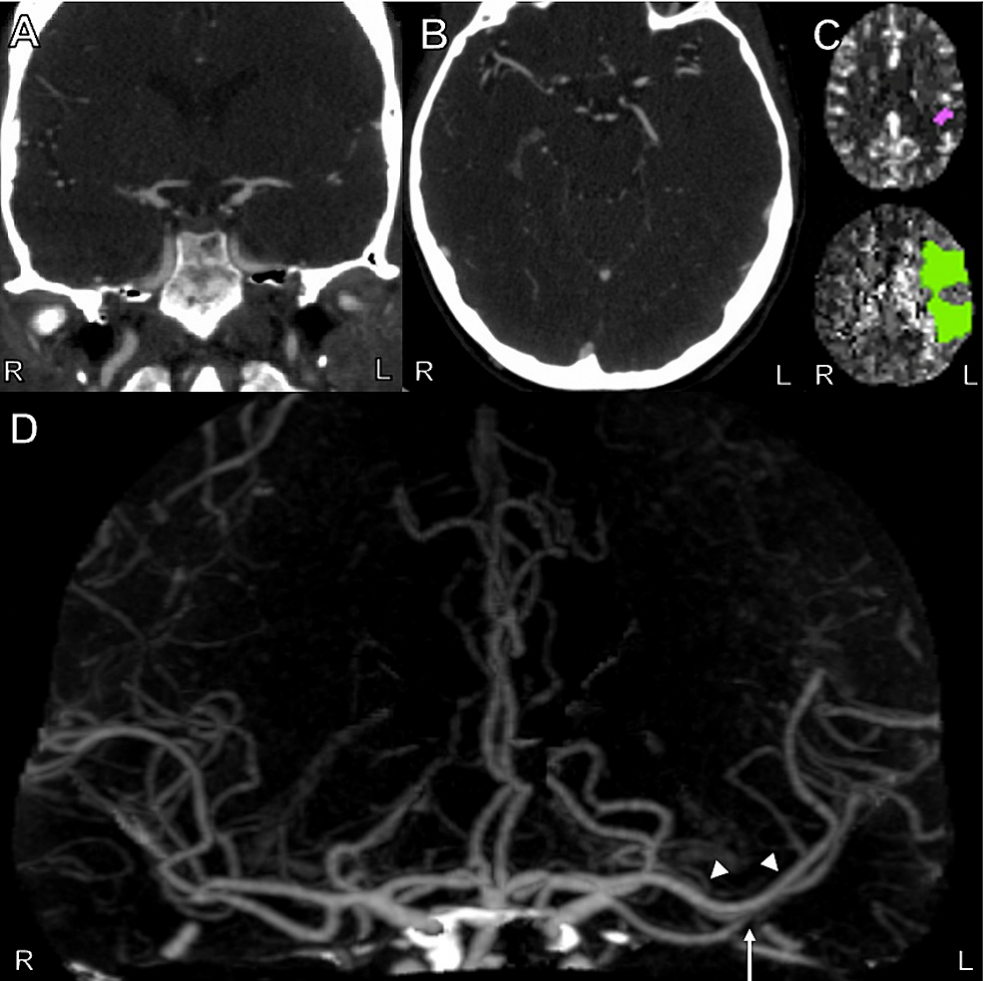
CTA and CT Perfusion Images of the Head from the Patient's Initial Work-up A: Coronal view of CTA scan of the head showing what appears to be normal flow through the right and left middle cerebral artery M1 segments. B: Axial view of CTA scan of the head showing what appears to be normal flow through the right and left middle cerebral artery M1 segments. C: Axial view of CT perfusion scan of the head showing core infarct (purple) and ischemic penumbra (green). D: Anteroposterior view of three-dimensional reconstruction of CTA of the head showing occluded left middle cerebral artery M1 segment (white arrow). The arrowheads point to a second MCA branch. Note the 'normal' appearance of MCA on the opposite (right) side. CTA, computed tomography angiography; MCA, middle cerebral artery.
